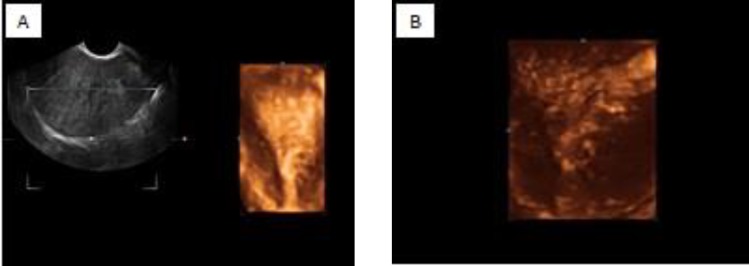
Three-dimensional ultrasound showing the complete infiltration of the junctional zone
